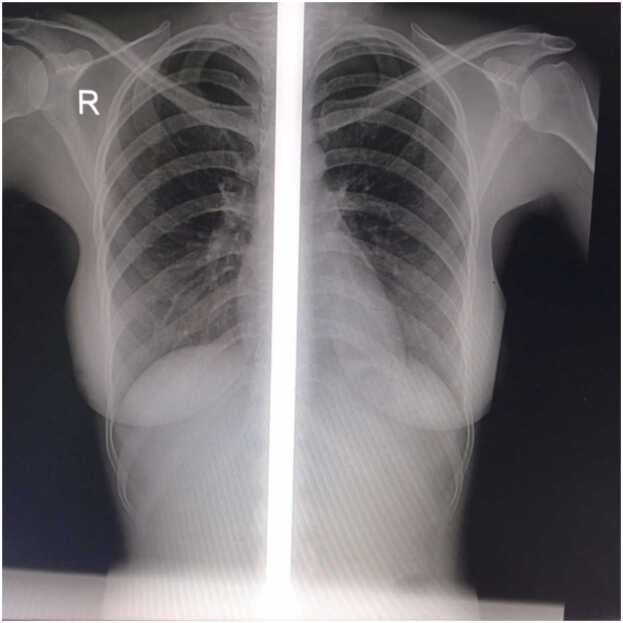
Normal chest radiography of the patient.
